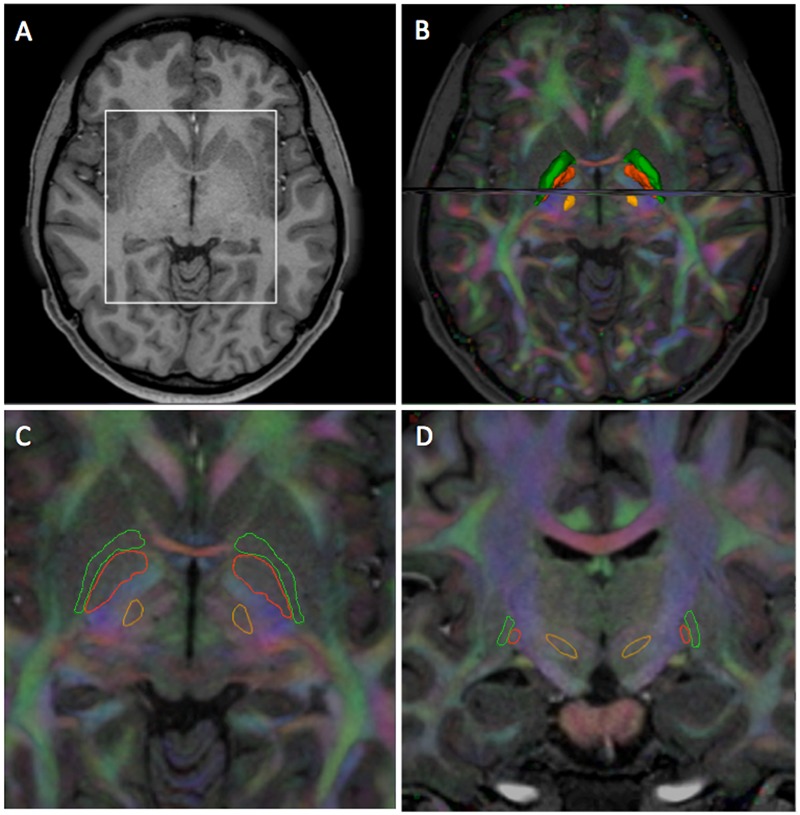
Segmentation of the STN, GPi, and GPe in HCP subject 100307. (A) Axial T1-weighted image. (B) 3D superior view of surface models of the STN (light orange), GPi (dark orange), GPe (green) with axial and coronal T1-weighted images overlaid on directionally encoded color (DEC) map. (C) Axial T1-weighted image overlaid on DEC map with outlined contours of the STN, GPi, and GPe. (D) Coronal T1-weighted image overlaid on DEC map with outlined contours of the STN, GPi, and GPe. The view presented in (C) corresponds to the ROI (white square) defined in (A). The view presented in (D) corresponds to the coronal image displayed in (B).
